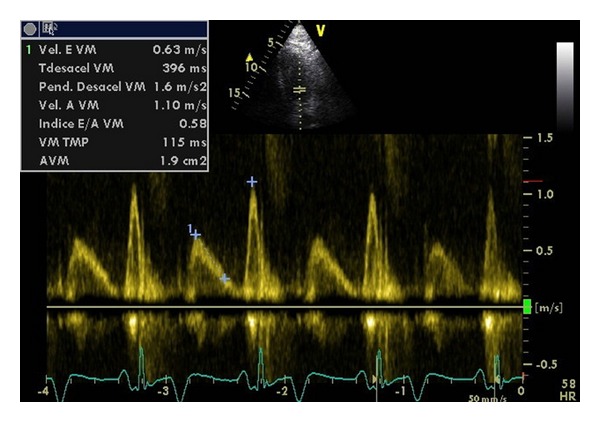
Diastolic dysfunction in a cirrhotic patient diagnosed during pretransplantation echocardiographic study (E/A ratio < 0.8, DTE > 240).
